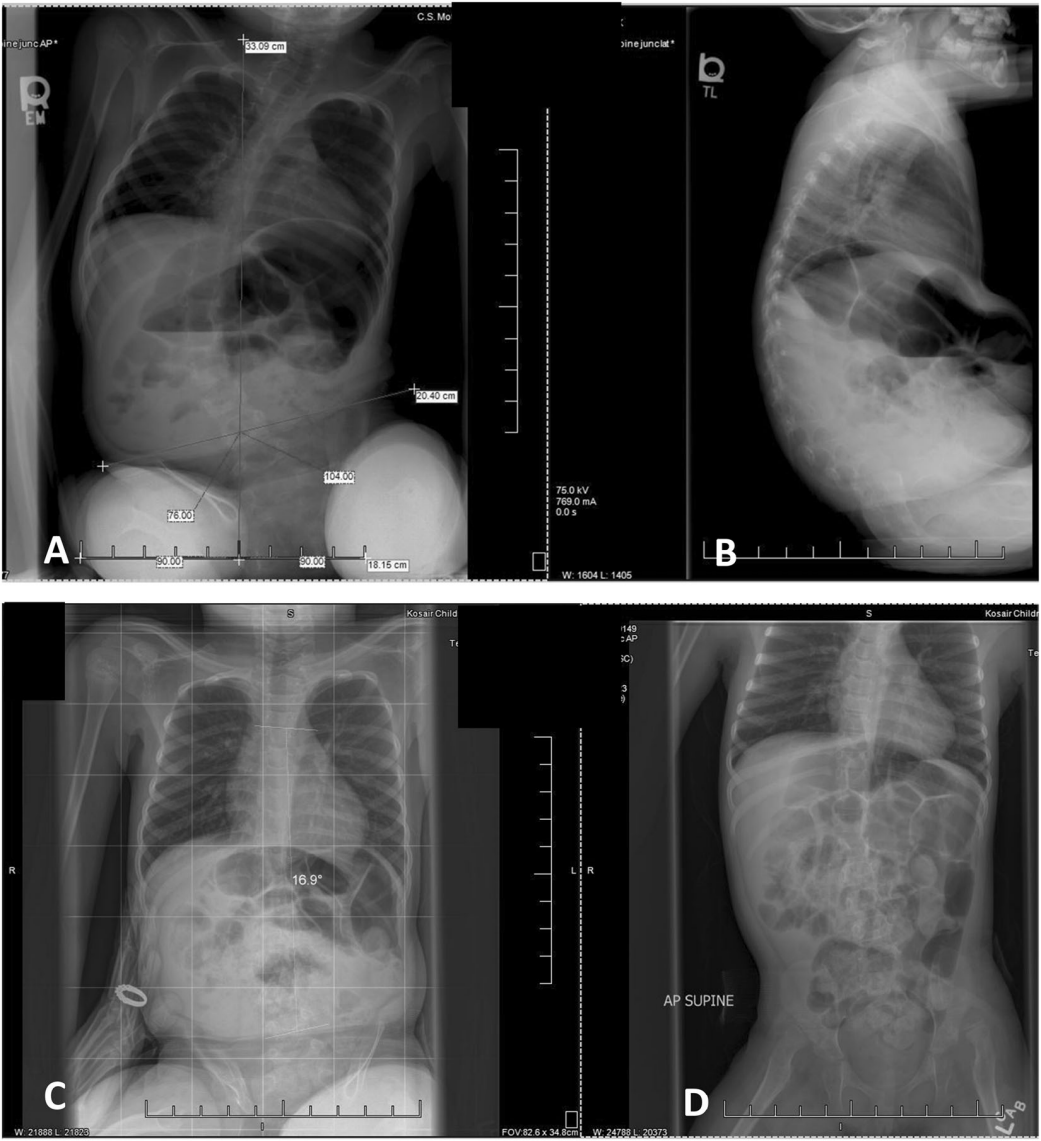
Spine x-rays diagnostic for scoliosis. Spine X-rays at age 6 years 1 month (a, b) and 6 years 5 months (c, d). At 6 years 1 month when imaged in sitting without request for active upright posture, she demonstrated a pelvic tilt of 14° and a spinal curve of 57° (a) as well as kyphotic posture (b). Four months later she was imaged again, this time she was verbally coached to “sit up tall” and use her arms to assist. She demonstrated a 16.9° spinal curve (c), the curve was also assessed in supine without external support (d) also resulting in a 17° curve.
